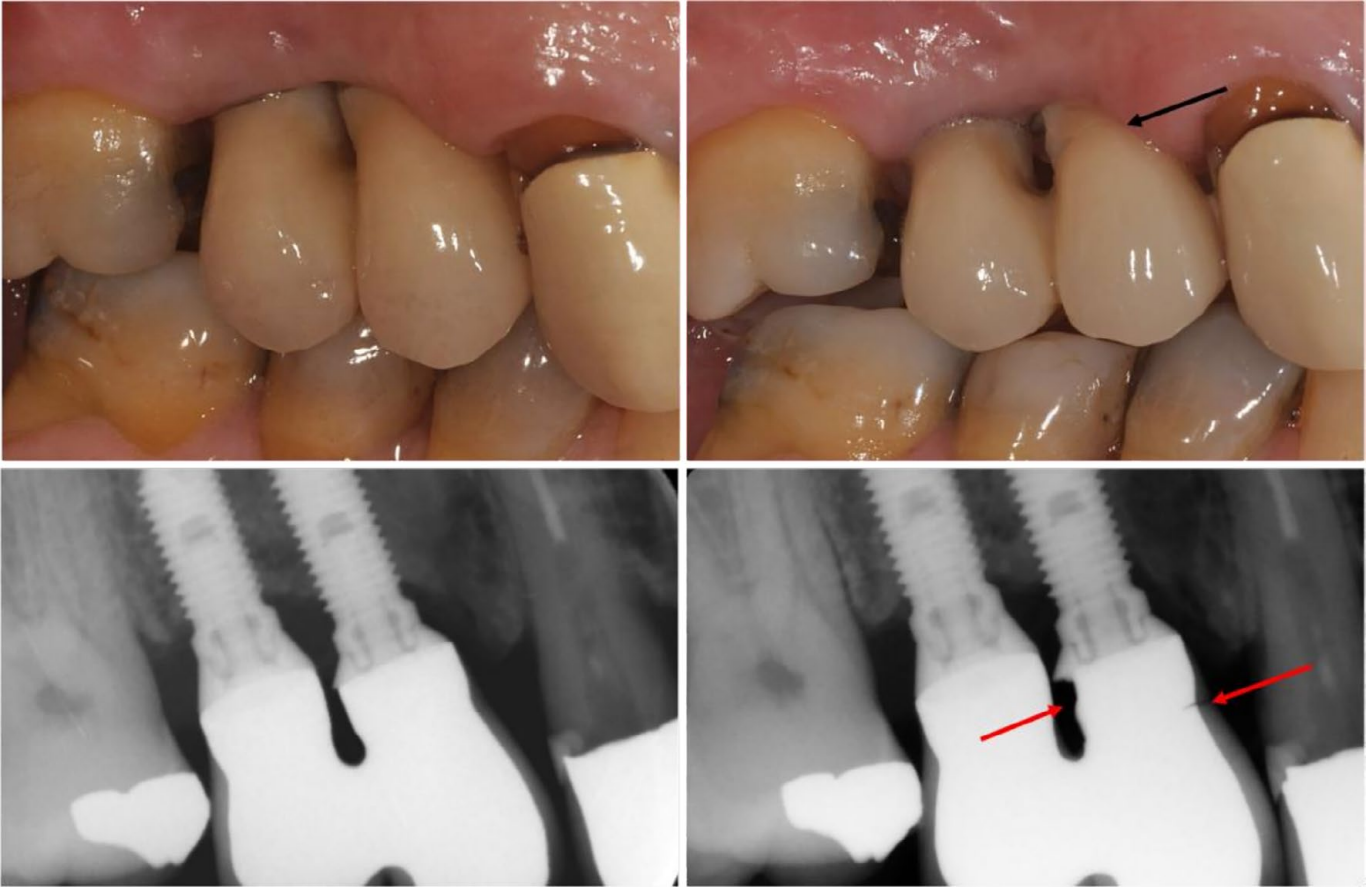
Clinical and radiographical images of a veneered zirconia (VZ) FDP at delivery (left). The upper images show the clinical view, while the lower images present the radiographs. The close proximity of the implants required proximal framework reduction for cleaning access. At the 1‐year follow‐up (right), a framework fracture is visible (arrows).
